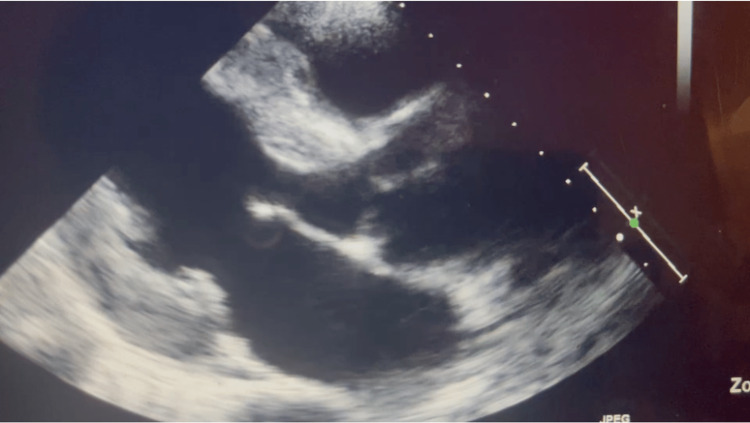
Repeat echocardiography noted interval improvement of systolic function with a left ventricular ejection fraction of 55-60%
